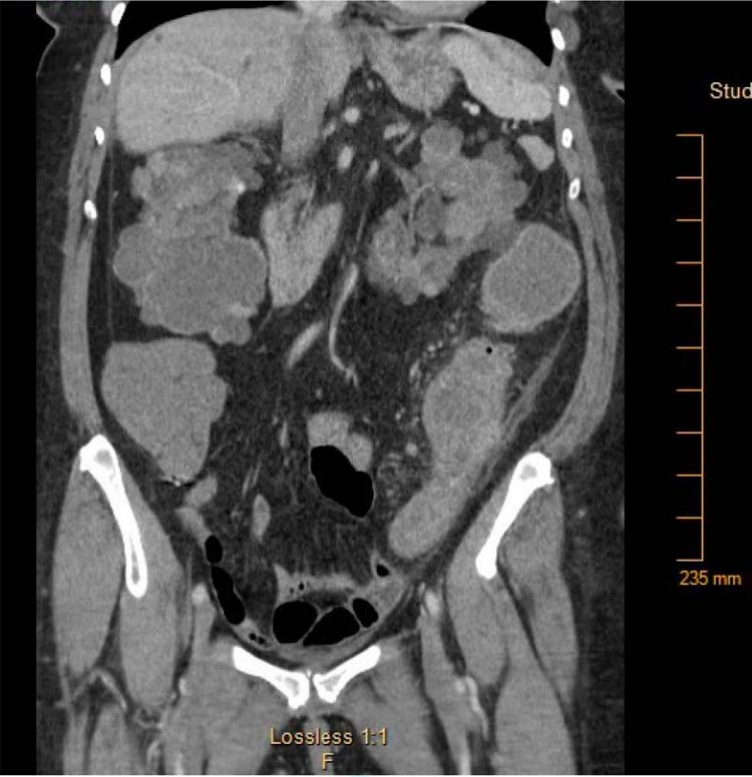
Computed tomography of the abdomen and pelvis showing focal narrowing of the sigmoid colon with proximal colonic dilatation.
